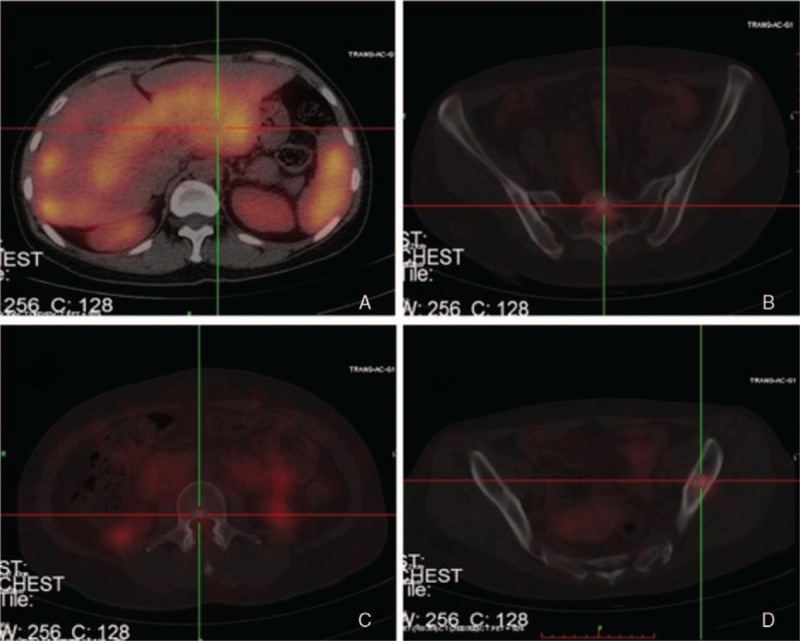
Somatostatin receptor scintigraphy. (A) Abnormal uptake in the liver; (B, C) Abnormal uptake in the lumbar spine; (D) Abnormal uptake in the left ilium.
